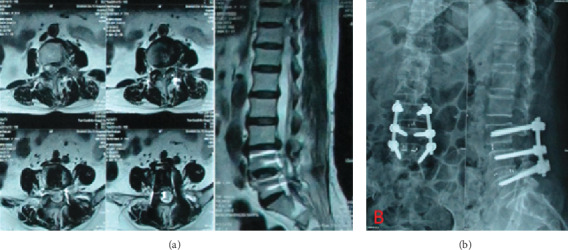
A case of PLIF surgery. (a) Preoperative Lumbar MRI images show L3/L4 intervertebral disk prolapse. (b) Postoperative frontal and lateral X-ray images.
